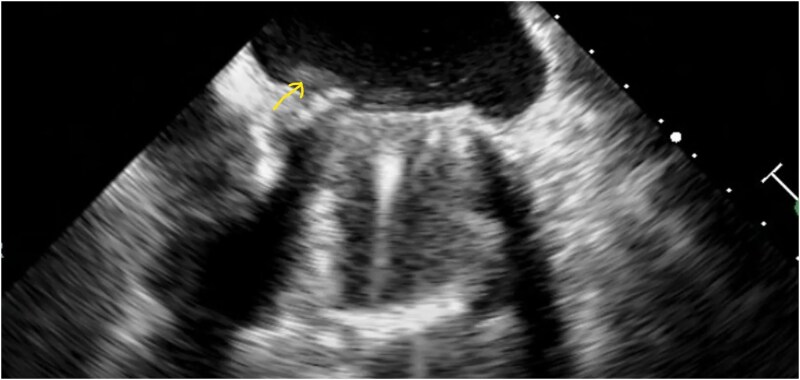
In the transoesophageal image, vegetation is observed on the mechanical mitral prosthetic valve.
